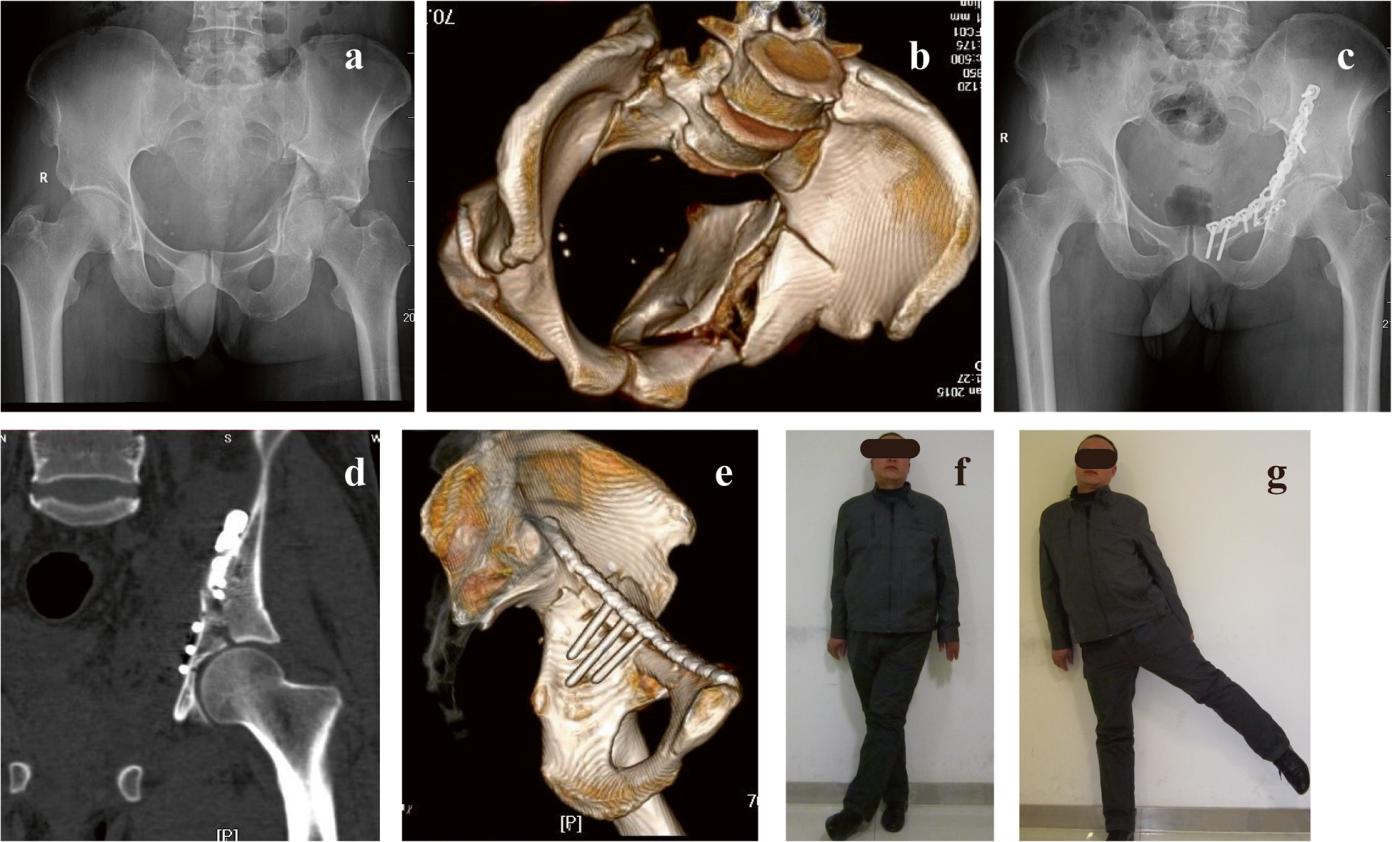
A typical case showing the use of the reconstruction plate for a displaced acetabular fracture. A 48-year-old man presented with both column fracture of the left acetabulum following a fall from a height. Preoperative AP (a) and 3D CT reconstruction (b) of the pelvis have confirmed the fracture pattern. On the 8th day after the injury, fixation was performed through the ilioinguinal approach by using a 12-hole reconstruction plate with 3 quadrilateral screws to control the medial displacement of the quadrilateral plate (c). Postoperative CT scanning image (d) and 3D CT reconstruction (e) showing the quadrilateral screws were located on the surface of the quadrilateral plate, and there was no one quadrilateral screw entering the hip joint cavity. At his 2 years of follow-up visit, the patient was symptom-free. The modified Merle d’Aubigné evaluation was scored as good (f, g)
